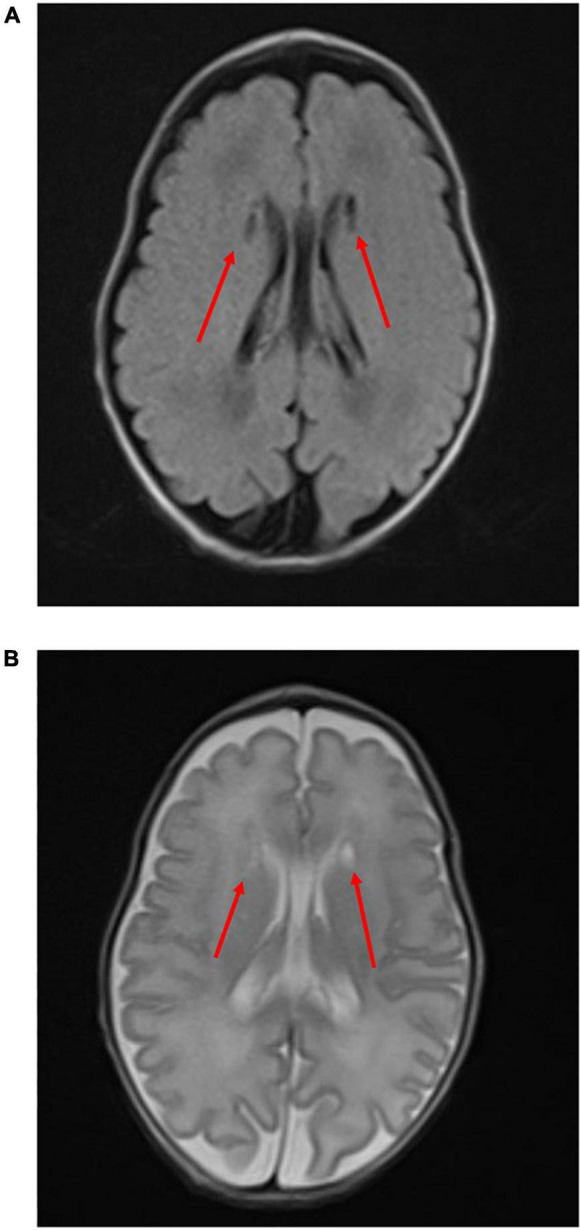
Brain MRI. (A) T2 flair: Red allows show bilateral cystic lesions. (B) T2-weighted: Red allows show bilateral cystic lesions.
